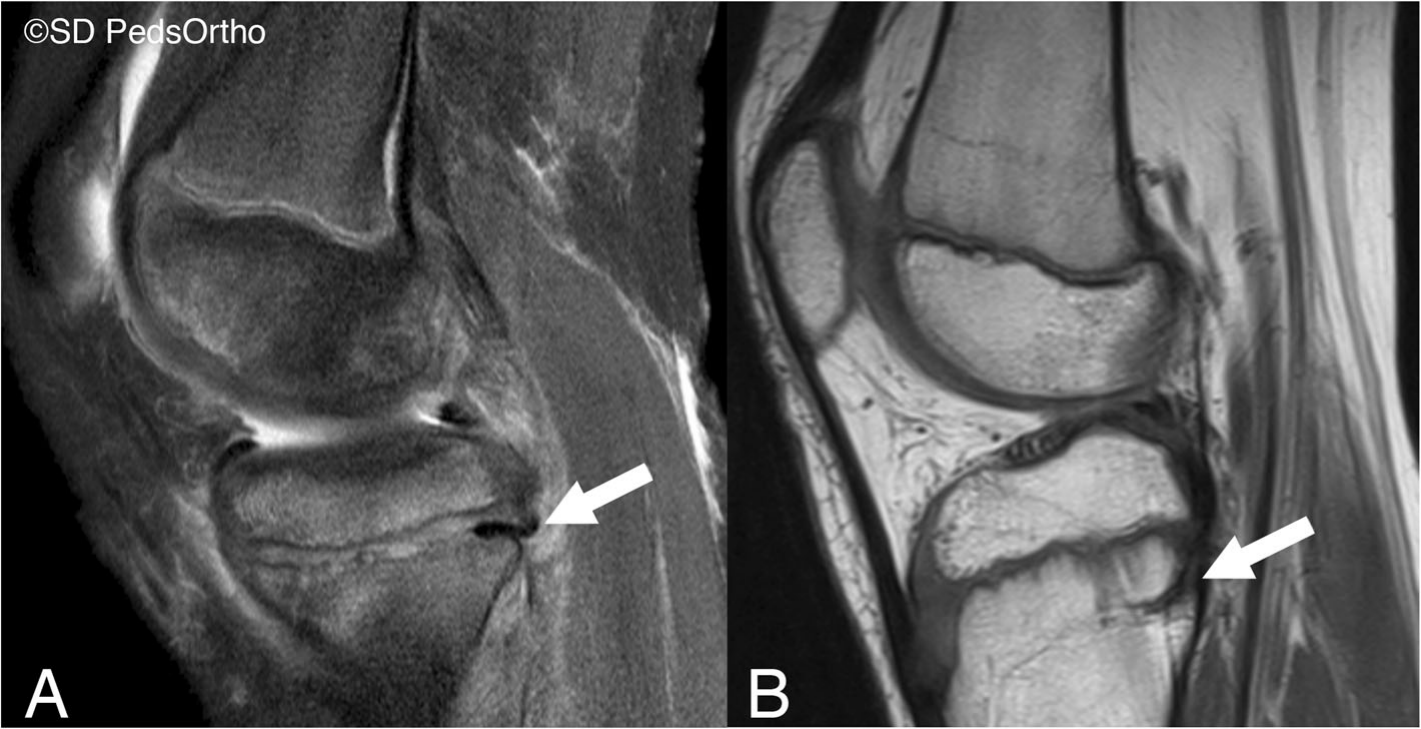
A 10 year old boy from our clinic practice. a Index MRI of the proximal tibial physis with evidence of periosteal tissue entrapment posteriorly (arrow); treated non-operatively. b 16-month follow-up MRI with continued growth, but evidence of periosteal remnant (arrow)
